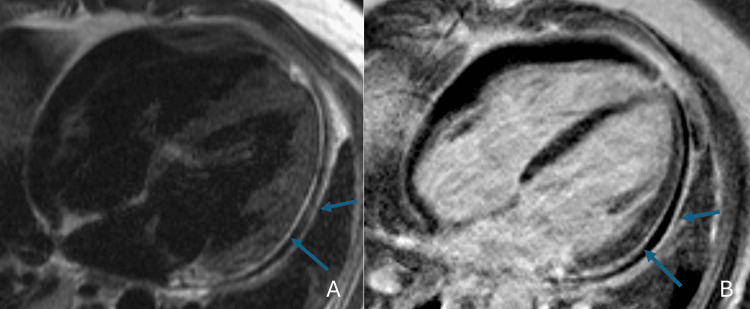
Cardiac MRI axial images (A) T2 image in the four-chamber view reveals subepicardial edema in the entire lateral wall and the adjacent pericardium (arrows). (B) Corresponding T1 PSIR post-contrast image reveals subepicardial late gadolinium hyperenhancement and the adjacent pericardium (arrows). There is a circumferential pericardial effusion. Findings are compatible with acute myocarditis and pericarditis. PSIR, phase-sensitive inversion recovery
